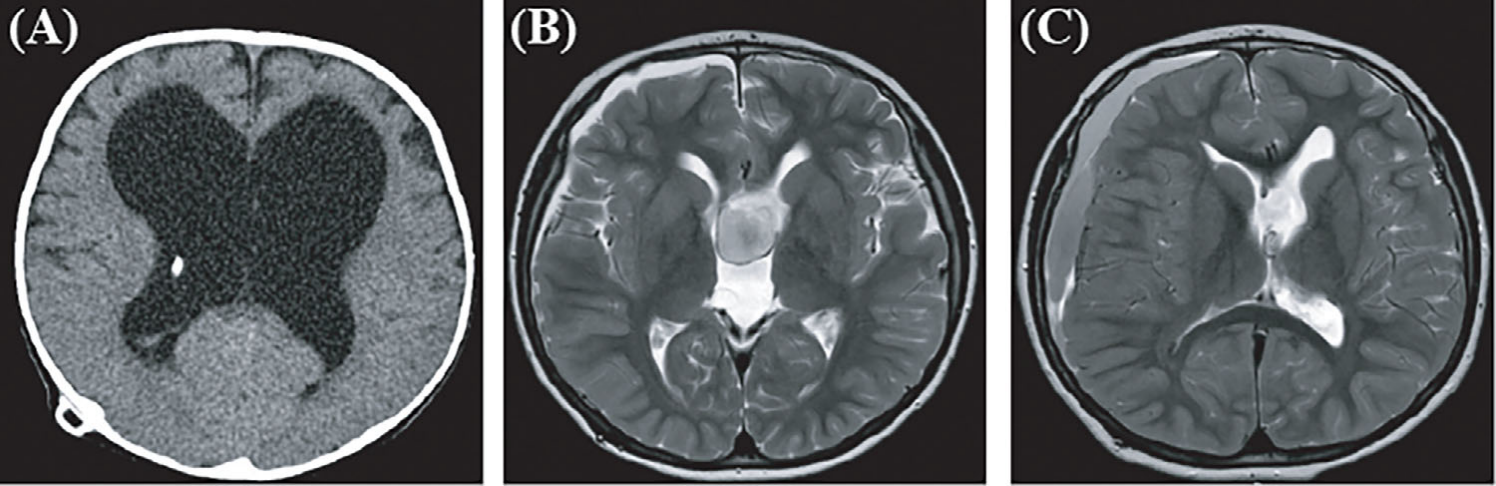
Image of patient 3. (A) Computed tomography (CT) scan showing hydrocephalus with a ventriculoperitoneal shunt; (B) Magnetic resonance imaging (MRI) T2 showing enlargement of the suprasellar cistern arachnoid cyst following shunt placement; (C) Disappearance of the suprasellar cistern cyst after ventriculostomy.
